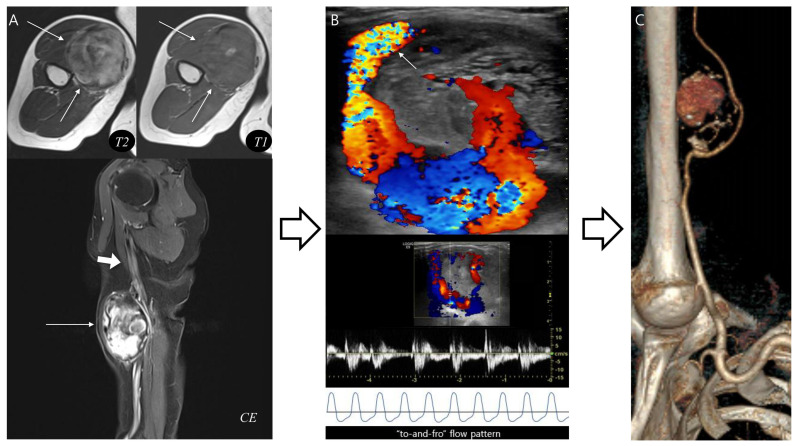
Pseudoaneurysm with neurovascular bundle involvement diagnosed without biopsy. (A) Axial T2-weighted and T1-weighted MR images demonstrate a hematoma-like lesions (thin arrows) with equivocal central flow void or absent inner peripheral high SI. However, neurovascular bundle involvement (thick arrow) in sagittal T1-weighted contrast-enhanced MR image raises suspicion for pseudoaneurysm. (B) Doppler ultrasonography reveals a characteristic “Yin-yang” sign with neck at the brachial artery (arrow), confirming a “to-and-fro” flow pattern. (C) CT angiography with 3D reconstruction demonstrates a pseudoaneurysm arising from the brachial artery, consistent with the MRI suspicion. This case highlights that recognition of distinct MRI features can guide appropriate diagnosis without unnecessary biopsy.
